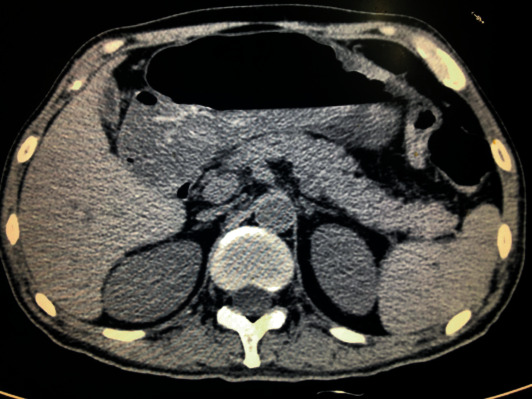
Stevens-Johnson syndrome. Routine upper abdominal CT to rule out acute pancreatitis of Patient 1, with a corresponding serum amylase of 663 U/L.
